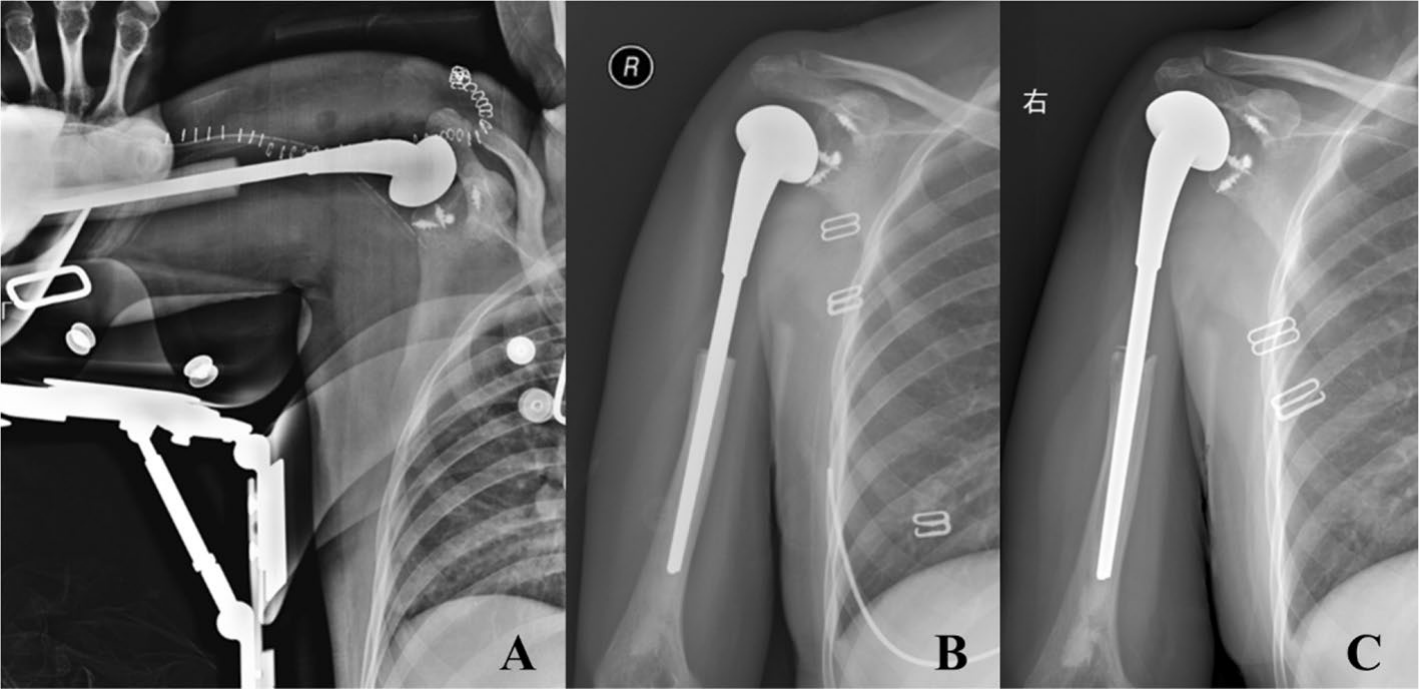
The postoperative radiography (A) showed 4 anchors were fixed in different directions (upper, lower, front and back) of the glenoid to restore the stability of the prosthesis. The radiography 3 months postoperative (B) and the radiography 104 months postoperative (C) showed the prosthesis was in stable position without obvious loosening and dislocation.
